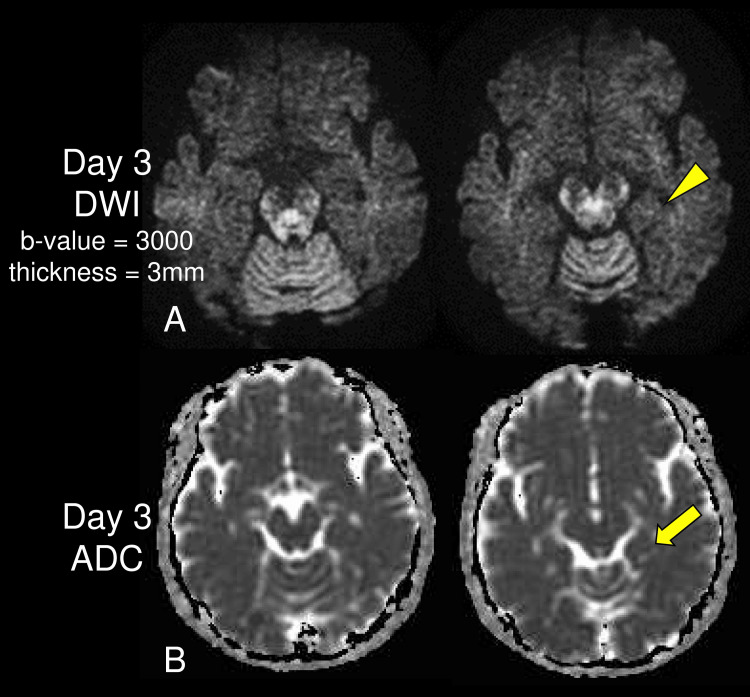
Magnetic resonance imaging on day 3 Diffusion-weighted images (DWI) on day 3 with a b-value of 3000 and thickness 3mm showed restricted diffusion in the left temporal lobes (arrowhead in A) along with corresponding apparent diffusion coefficient (ADC) images (arrow in B).
